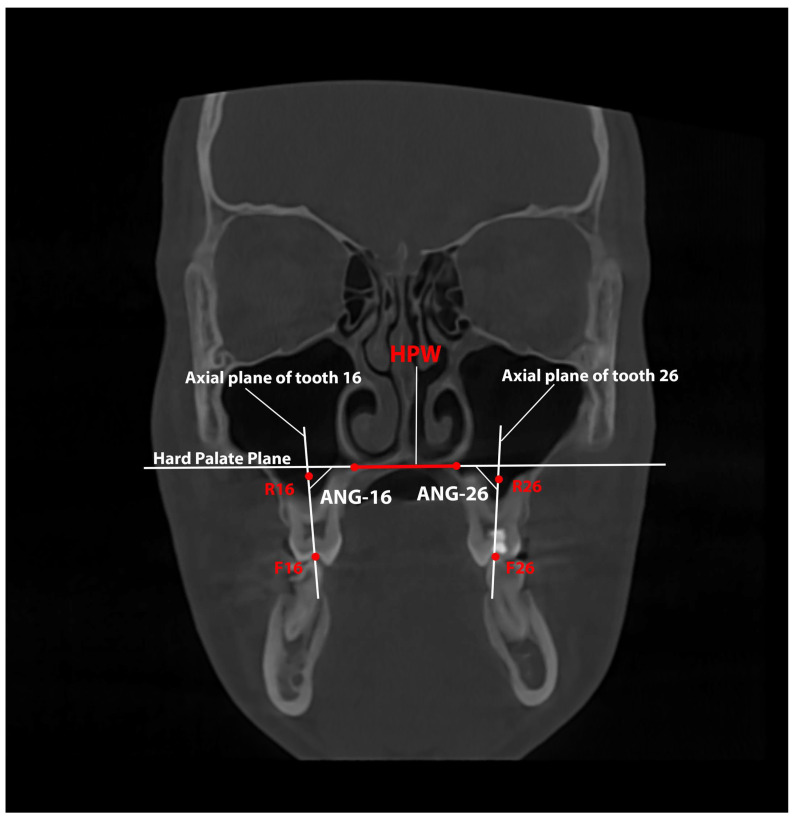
Assessment of the inclination of the upper first molars relative to the plane of the hard palate measured on a CBCT coronal slice. The Hard Palate Width (HPW) is indicated with the red line and the corresponding plane is indicated in white. The red dots indicate the points (R16, R26, F16, and F26) that define the axial plane of the upper first molars (represented by the two vertical white lines).
